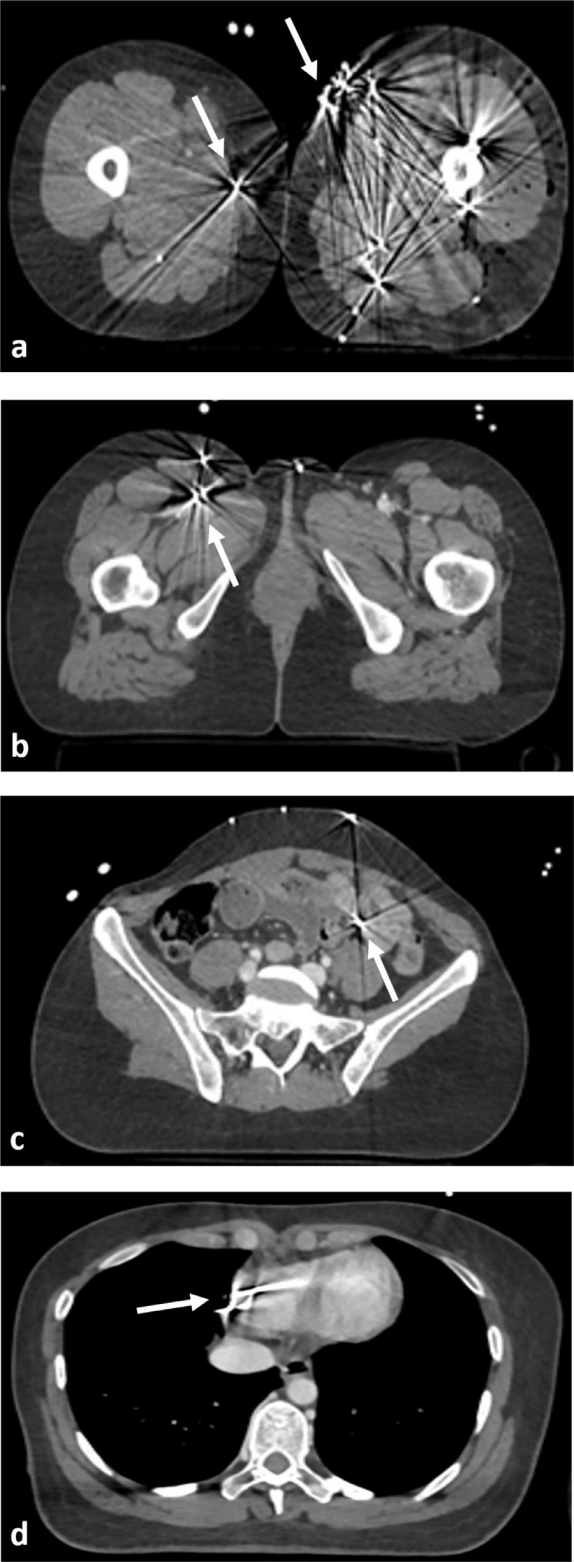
Axial CT slices showing multiple pellets (white arrows) within both thighs (A), in the right groin adjacent to femoral vessels (B) and inside the peritoneal cavity (C). At the level of the chest (D), axial slice showing metallic foreign body (white arrow) at the right ventricular wall for which exact location could not be assessed due to metallic and cardiac motion artifacts.
